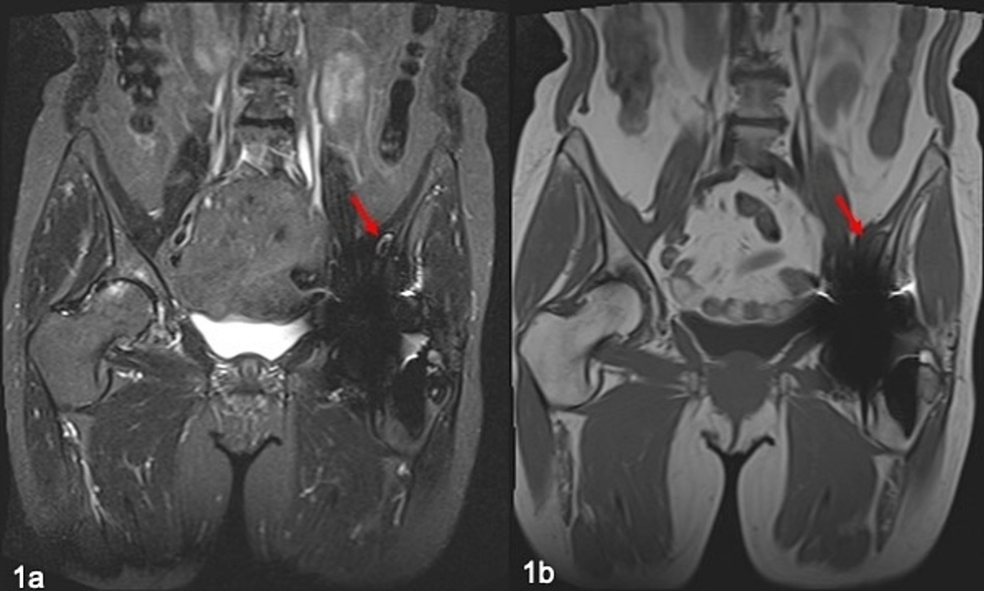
MRI of pseudotumour (red arrows) following metal-on-metal left hip arthroplasty in 2011. (a) Coronal STIR; (b) coronal T1. MRI: magnetic resonance imaging; STIR: short TI inversion recovery
